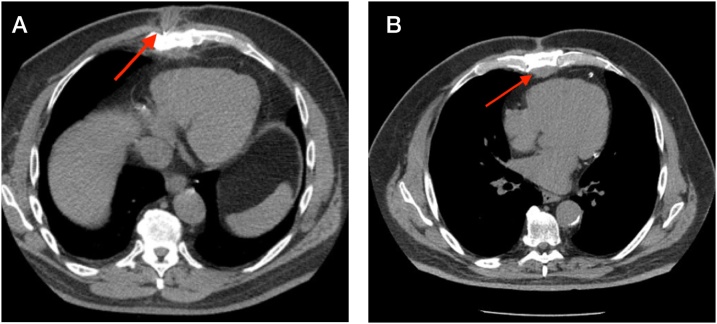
Axial thoracic computed tomography (CT) image showing soft tissue density anterior to the sternum (red arrow, panel A); subsequent CT image showing soft tissue density posterior to the sternum (red arrow, panel B).
